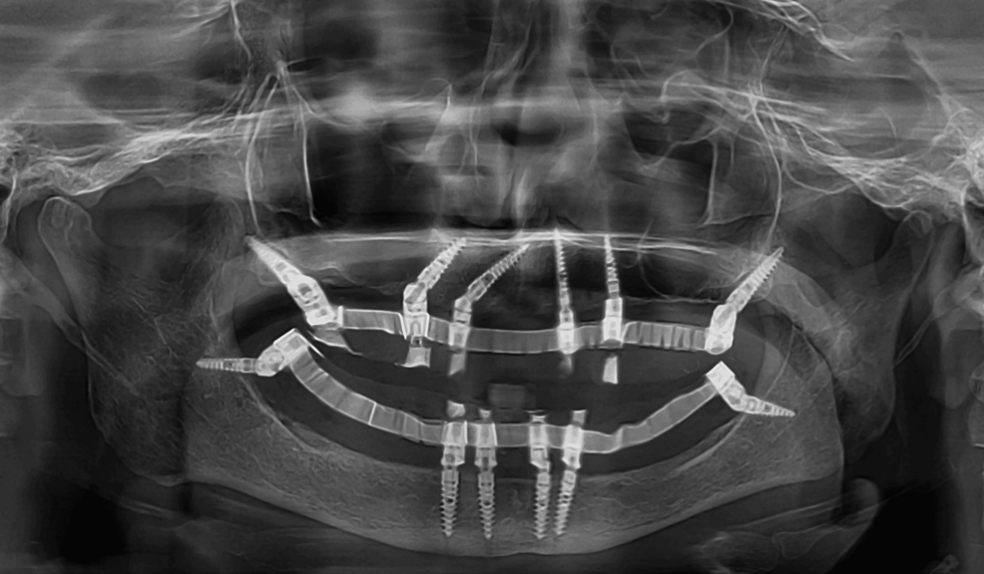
Orthopantomogram of the patient after provisional prosthesis placement
